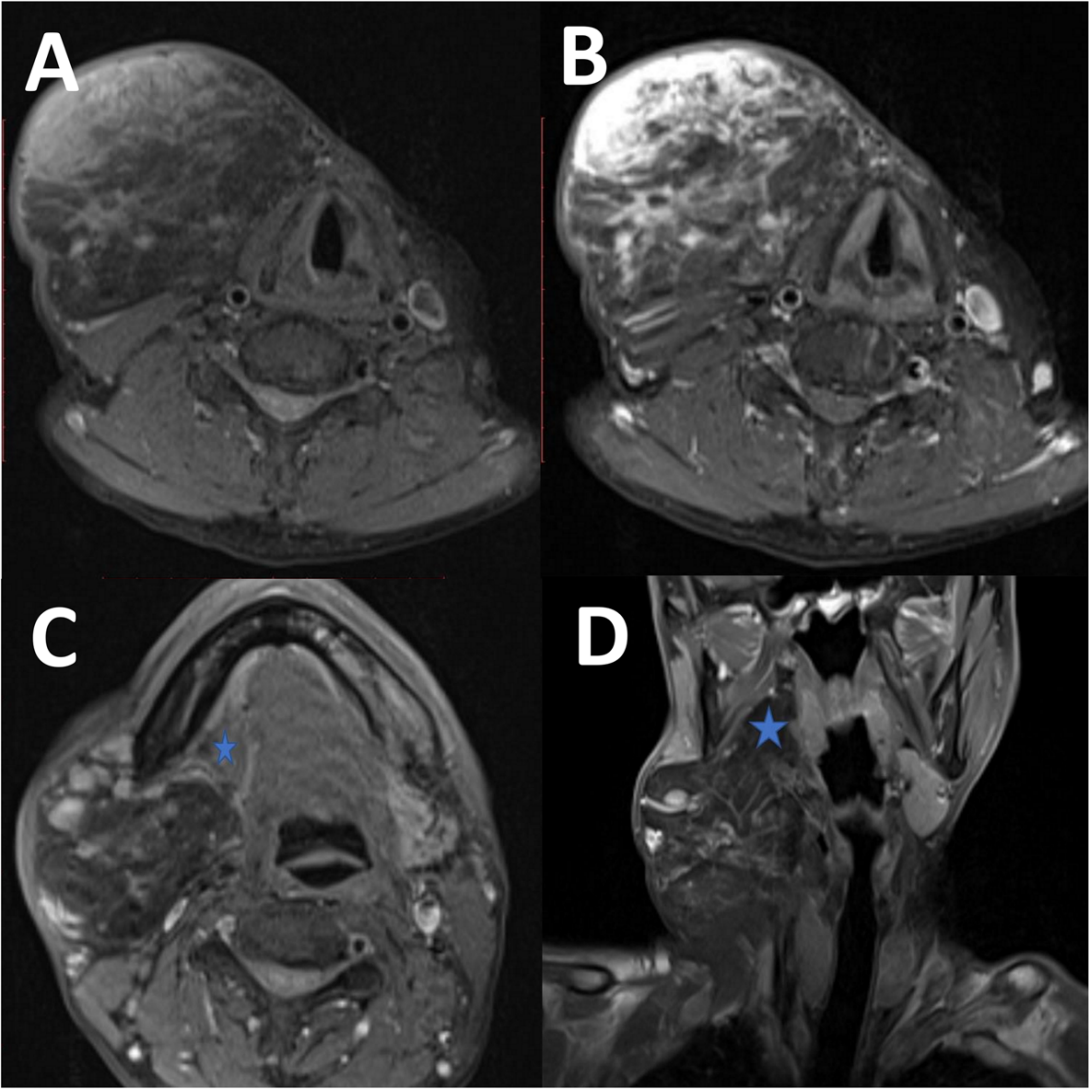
a Axial MRI on T1-weighted fat suppression sequence showing hyperintense soft tissue component in the anterior portion of the mass with nodular irregular septation within the mass. b Post contrast axial MRI on T1-weighted fat suppression sequence showing avidly enhancing soft tissue component in the anterior portion of the mass with enhancing nodular irregular septation within the mass. c Axial MRI on T1-weighted fat suppression sequence showing hyperintense soft tissue component in the anterior portion of the mass with nodular irregular septation within the mass. The right submandibular gland (blue star) is being displaced anteriorly by the mass. d Coronal MRI on T1-weighted fat suppression sequence showing the mass insinuating into the right parapharyngeal space from the right margin of the right anterior neck, elevating the right pterygoid muscles (blue star)
